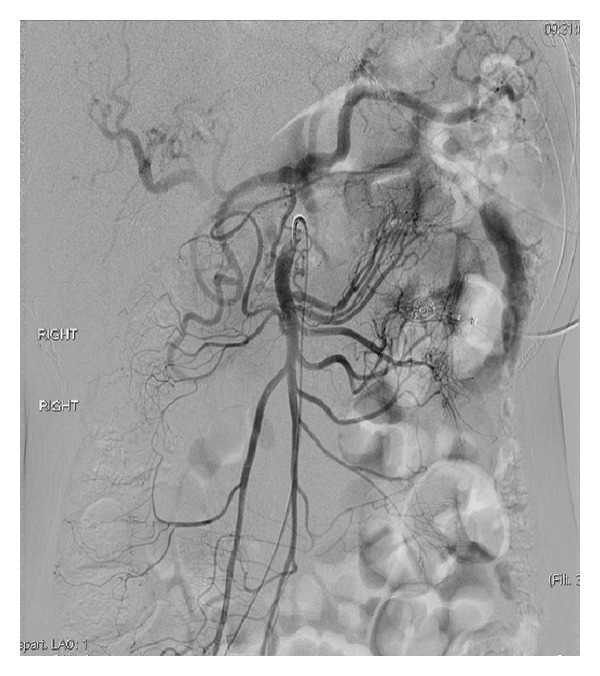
CT angiogram showing increased retrograde flow through the collaterals from superior mesenteric artery to celiac artery.
